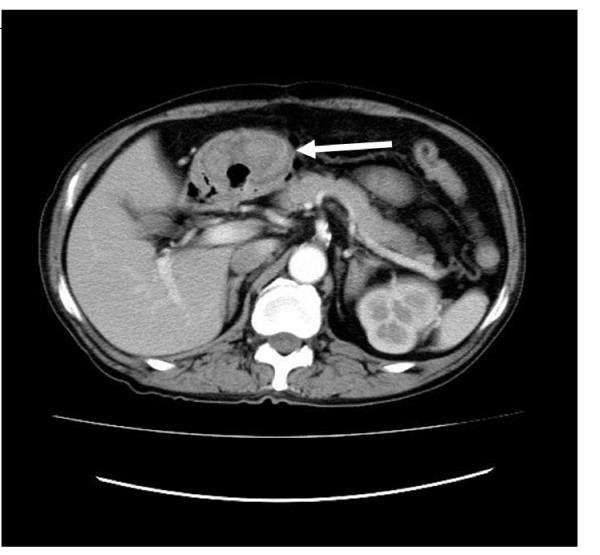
An abdominal computed tomography (CT) scan showing a heterogeneous mass (3 cm × 4 cm) located in the lower part of the stomach body. The tumor is indicated with an arrow.
